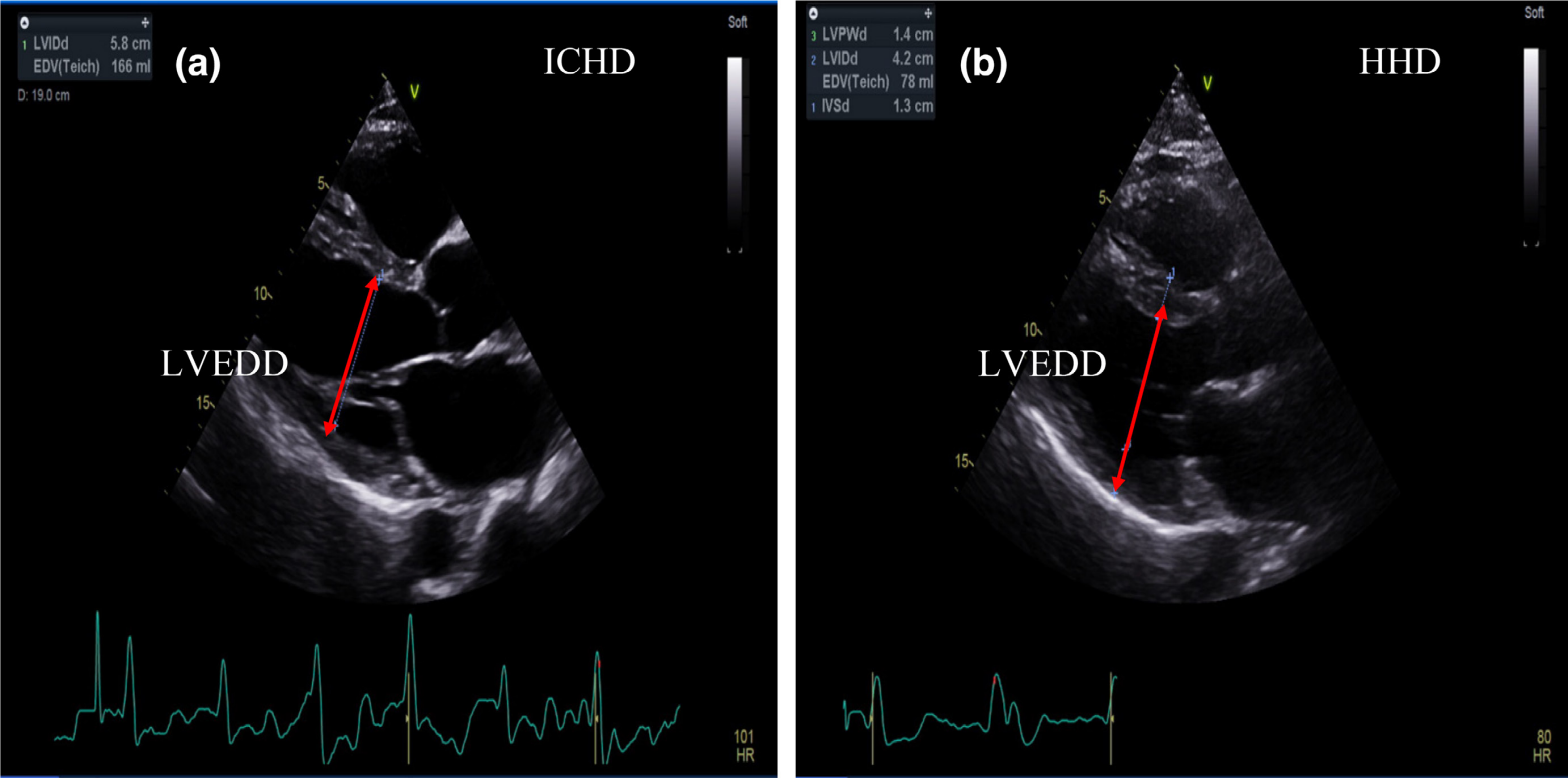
Linear measurements of left ventricular (LV) dimensions in systole and diastole, assessed through the use of transthoracic echocardiography in an adult with end‐stage kidney disease (ESKD) who was receiving home hemodialysis (HHD) (Plot a) and an adult with ESKD who was receiving in‐centre hemodialysis (ICHD) (Plot s). A significantly larger (*p* = 0.03) LV end diastolic diameter can be seen in the adult receiving HHD.
